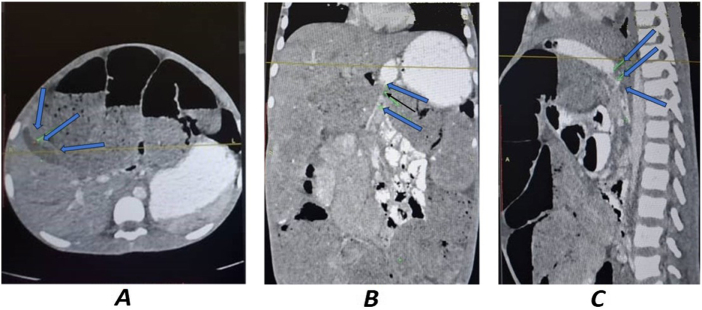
Gallblader and duodenal compression. (A) CT-scan axial view (gallblader compression, large bowel loops edematous and dilated). (B) CT-scan coronal view (duodenal compression). (C) CT-scan sagittal view (duodenal compression, large bowel loops edematous and dilated).
